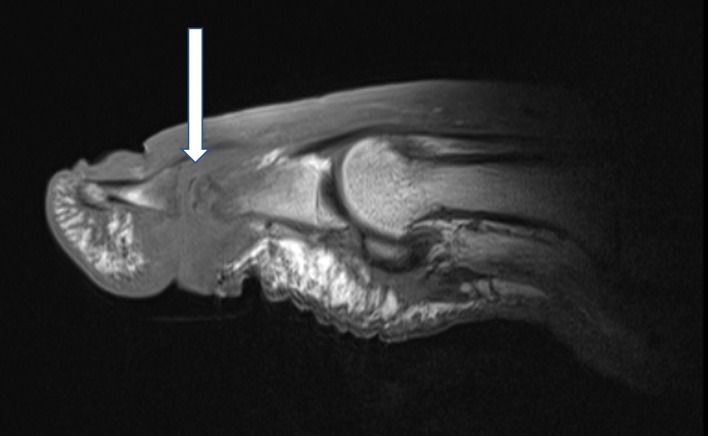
T1-weighted sagittal images of a right first
